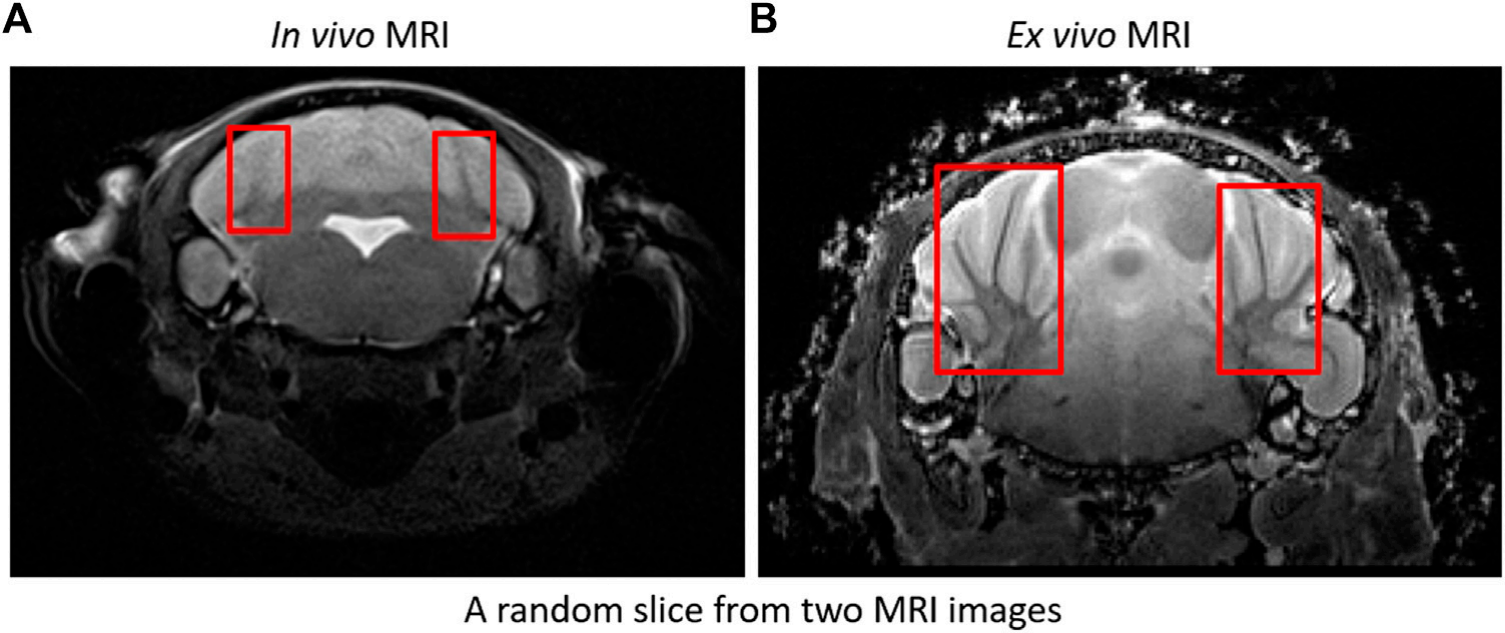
In vivo* and *ex vivo* MRI images: Sample *in vivo*
